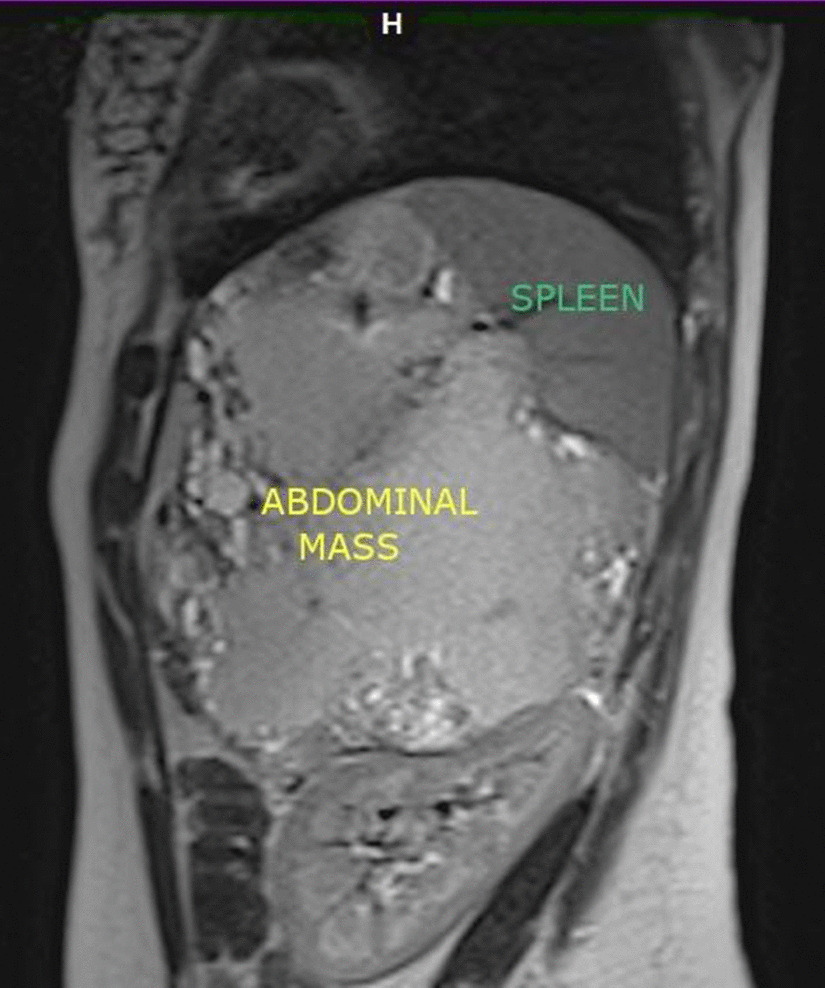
CT scan sagittal view showing a splenic hydatid cyst of 16 cm diameter
